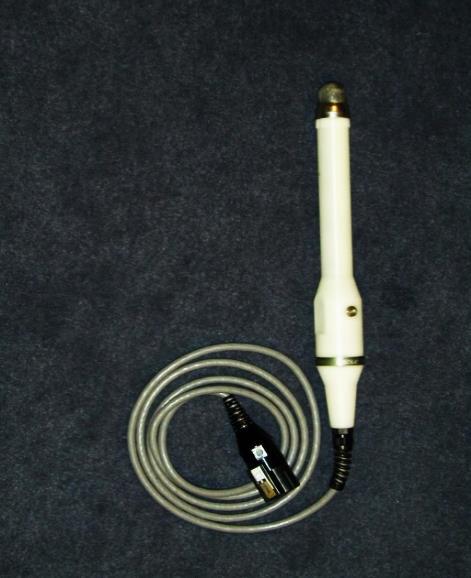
The First Elscint Transvaginal Ultrasound Probe.
